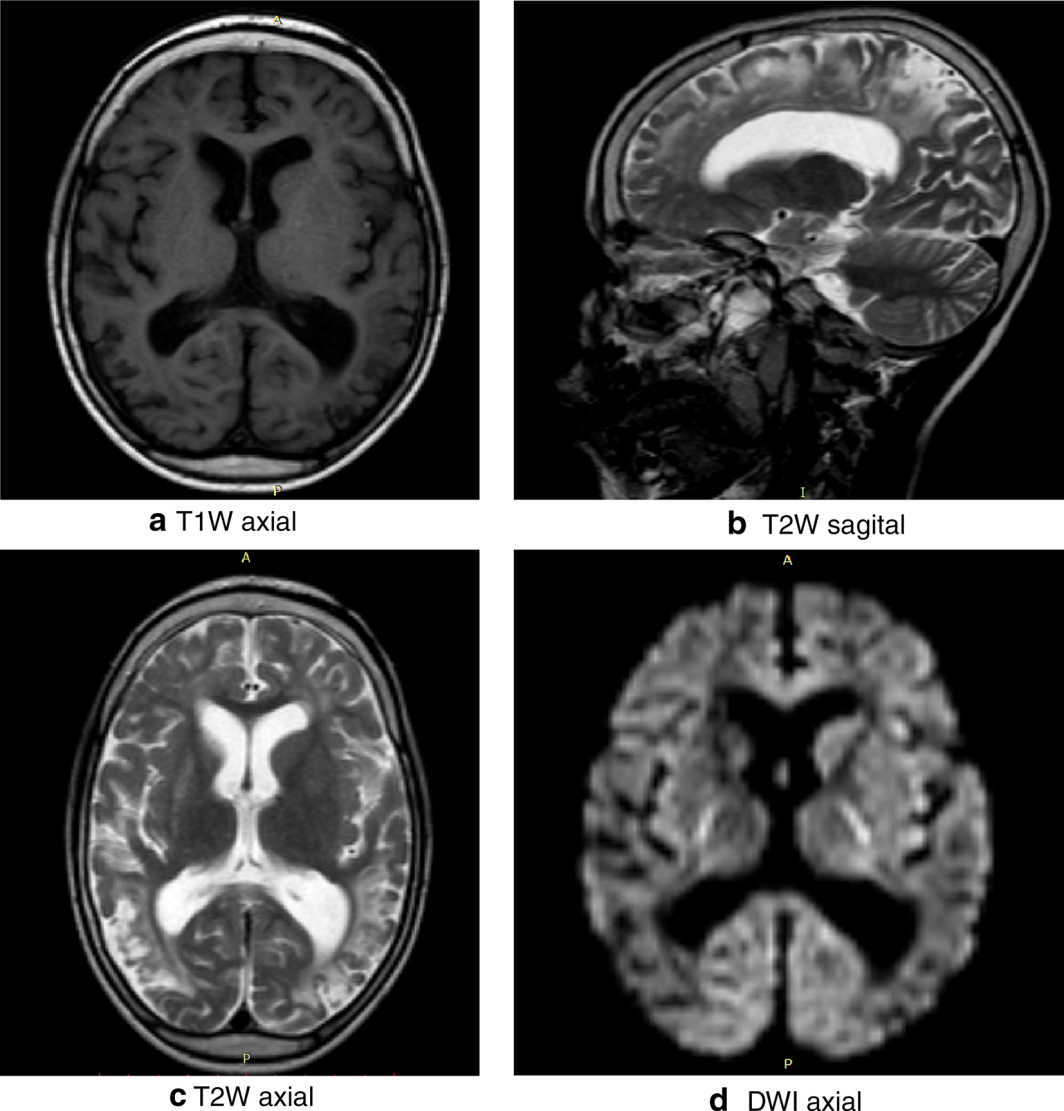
This is a set of brain MRI images of a 5 years old boy who suffered cerebral malaria at the age of 3 years and 3 months. On discharge he had severe neurological sequelae with motor, visual, hearing, and speech deficits and later developed epilepsy. At the time of imaging 20 months later, the visual, speech and motor difficulties had markedly improved but he had developed conduct problems and attention deficit and hyperactivity disorder with violent and aggressive behaviour. The images show generalized widening of sulcal spaces and sylvian fissures in both cerebral hemispheres. There are numerous bilateral T1 W hypointense foci showing confluence in some areas (a); hyper-intense foci in the *grey mater* and sub cortical regions of the frontal occipital and temporal lobes on T2 W (b and c) and fluid fluid-attenuated inversion recovery (FLAIR) images. There was no restriction of water diffusion on DWI, (d)
